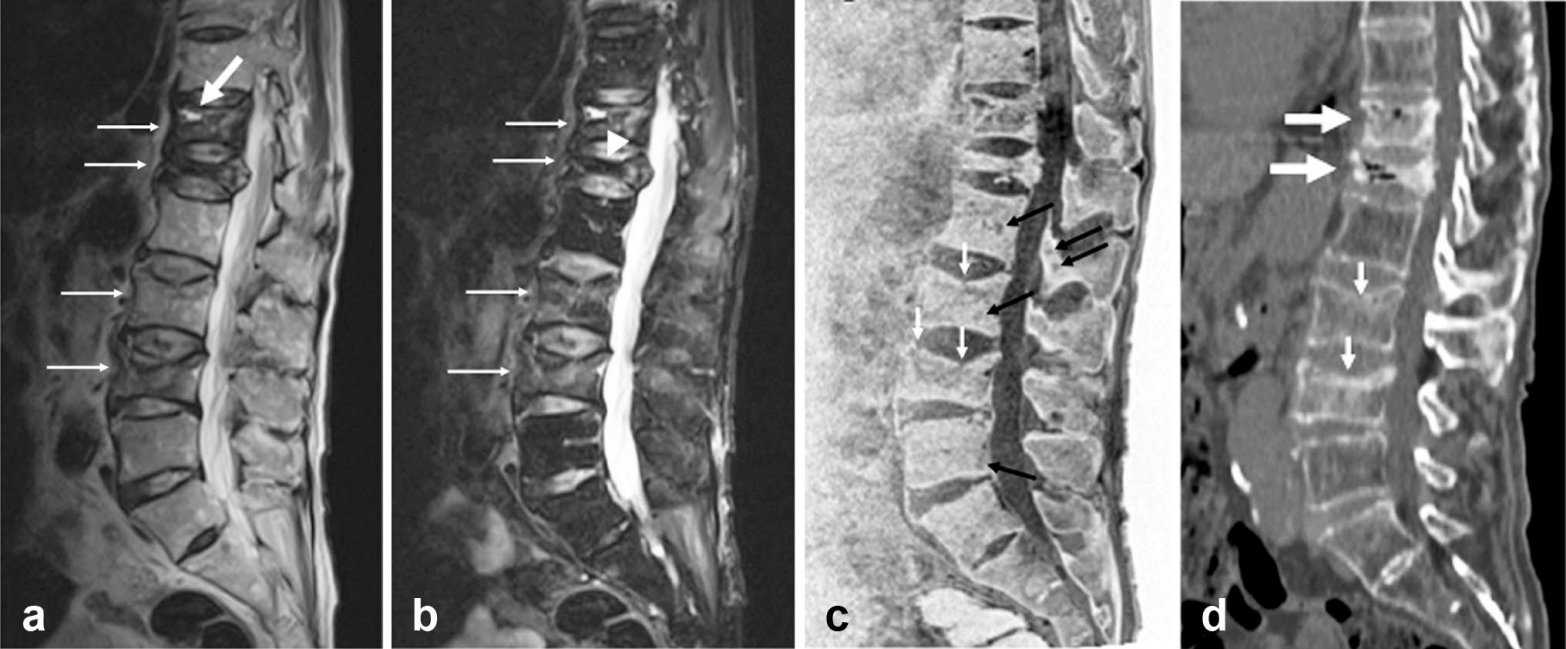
Recent pathologic compression fractures associated with old compression fractures. Sagittal T2-weighted MR image a shows compression fracture of Th11, Th12, L2, and L3 in a 77-year-old male patient with lung cancer (small arrows). Fluid sign is noted in the Th11 fracture (large arrow). Sagittal fat-suppressed T2-weighted MR image b shows the multiple fractures (arrows) and hypointensity in most parts of Th12, indicating old fracture (arrowhead). The hyperintensity of L2 and L3 suggests acute fracture. Part of Th11 also shows hyperintensity, suggesting recurrent acute fracture. On sagittal MR bone image c, the collapsed Th12 shows hyperintensity due to sclerosis. A tiny disruption of the cortex of L2 and L3 suggests a recent fracture (white arrows). Small hypointense lesions are probable metastatic foci from lung cancer (black arrows). Sagittal CT image d shows a sclerotic change of Th11 and Th12 as well as air indicating old fractures (large arrows). It also shows fracture of L2 and L3 with disruption of the cortex that is compatible with recent fracture as suggested on MR bone imaging (small arrows)
